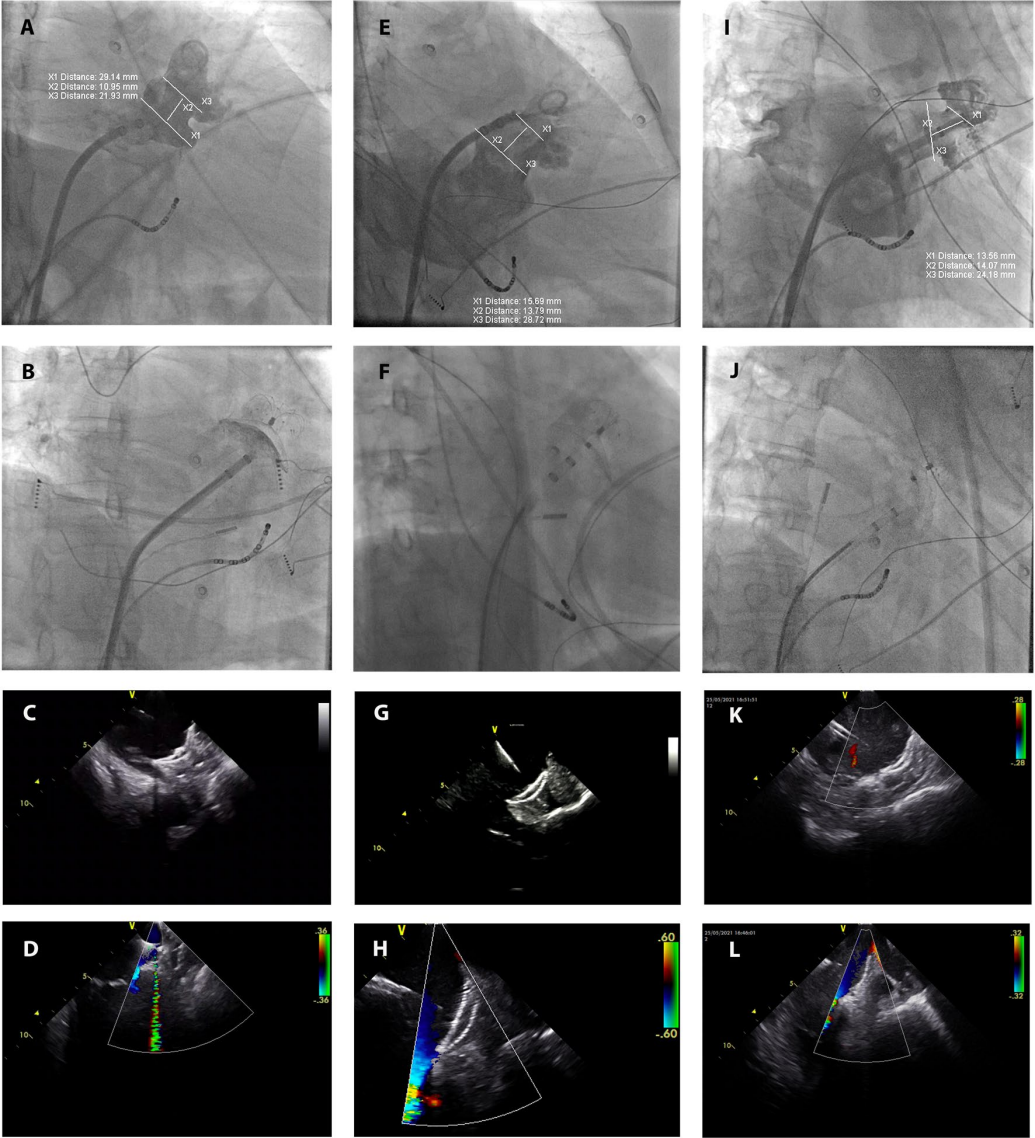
The small-umbrella LAmbre device anchored in the branch for multi-lobe LAA. A–D Case 1 using a small-umbrella LAmbre device. E–H Case 2 using a small-umbrella LAmbre device. I–L Case 3 using a small-umbrella LAmbre device. A, E, I LAA angiography. B, F, J Complete sealing of the LAA by the device. C, G, K Intracardiac echocardiography showing LAA occlusion by the LAmbre device. D, H, L Intracardiac echocardiography showing no peri-device leak. LAA: left atrial appendage; X1: diameter of the landing zone; X2: length of the central flexible waist; X3: diameter of the LAA orifice
